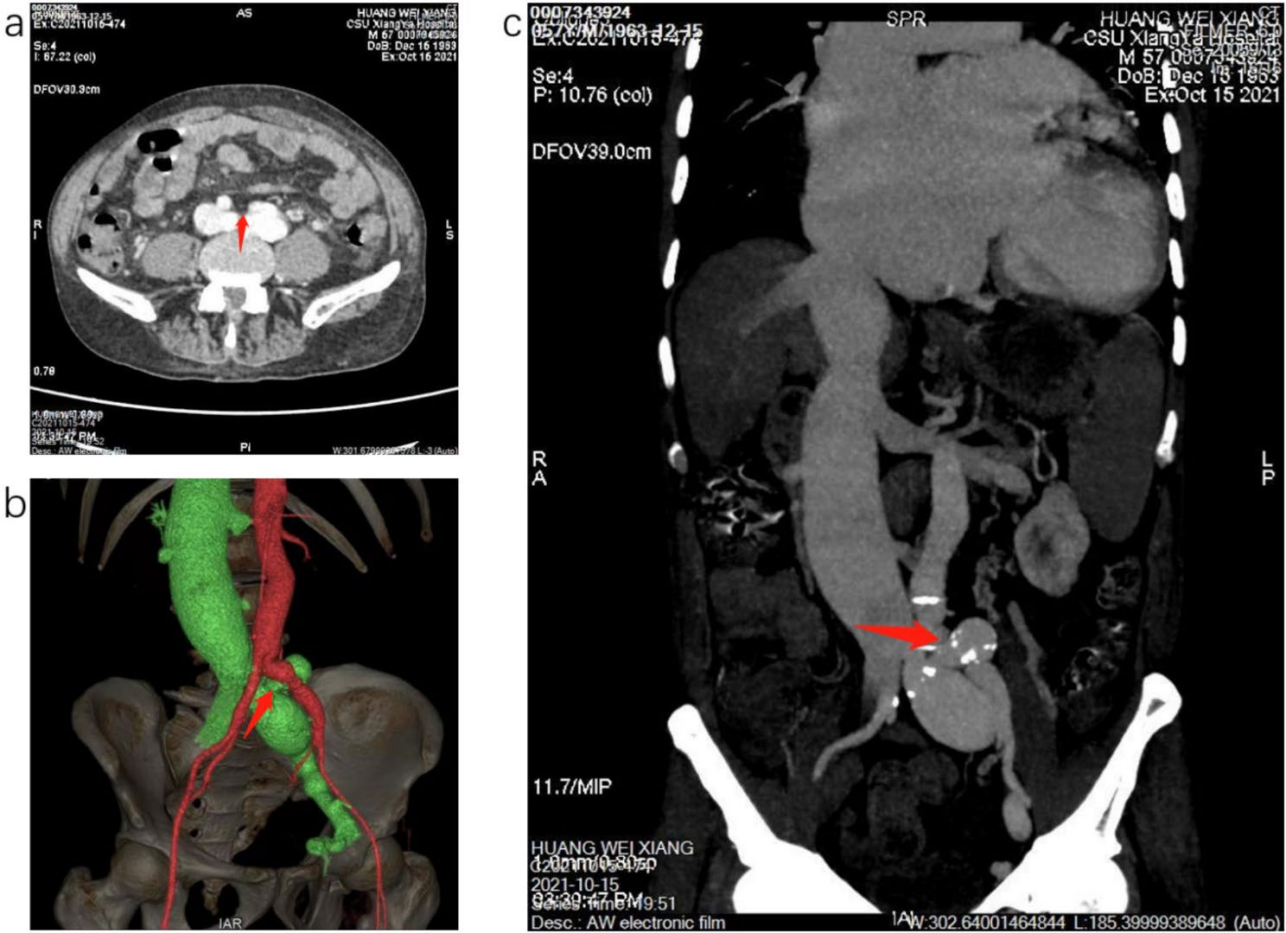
Computed tomography angiography (a axial, b coronal, and c 3D volume-rendering image) showing left iliac arteriovenous fistulae (marked by red arrows)
